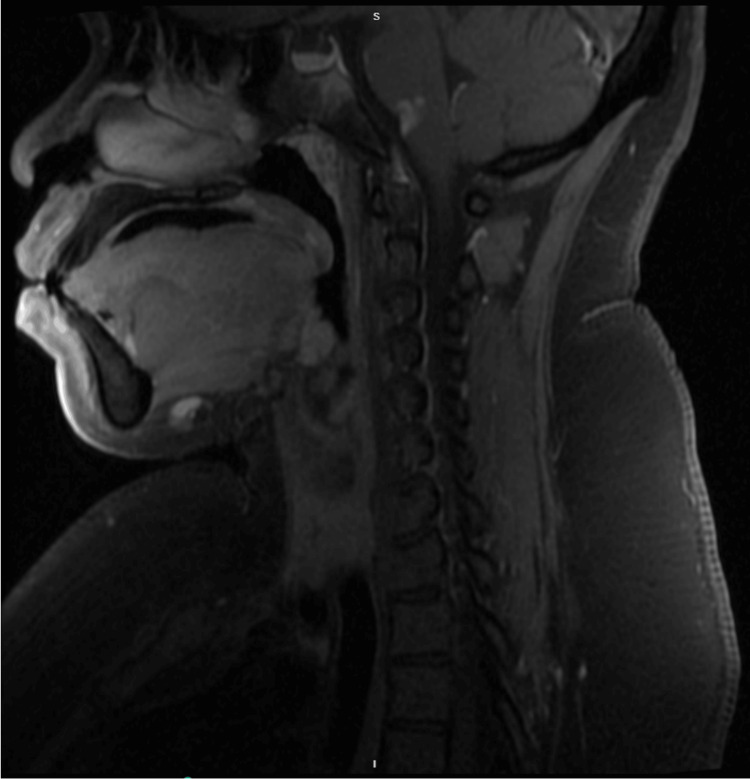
MRI of cervical spine showing no abnormal enhancement
